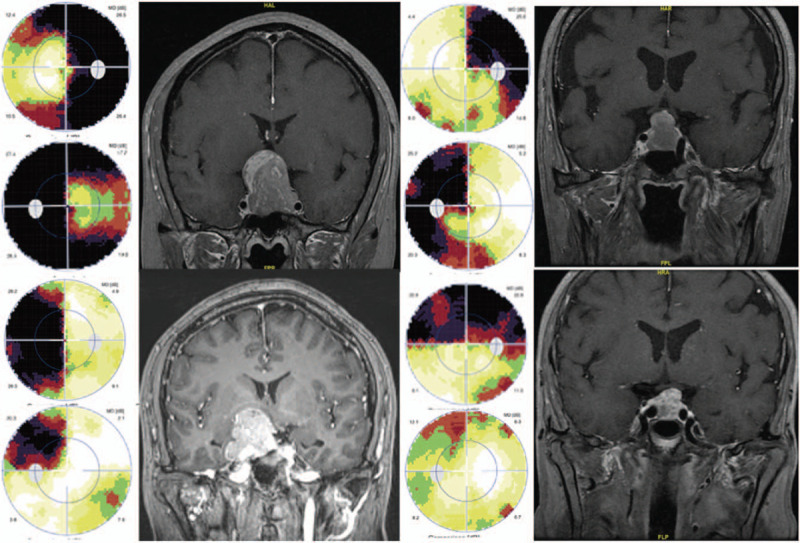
Visual field and MRI of four patients with pituitary adenoma. Top-left: Case 1. A 53-year-old woman with NFPA at diagnosis. Visual field: bilateral temporal hemianopsia breaking through the vertical midline. Contrast-enhanced MRI shows that the pituitary dumbbell-shaped mass with a clear boundary is significantly enhanced, with a size of 2.7 × 2.6 × 4.0 cm. The mass protrudes to the sella and compresses the optic chiasma. Top-right: Case 2. A 70-year-old man with NFPA at diagnosis. Visual field: bilateral superior temporal quadrant visual field defect and breakthrough to the inferior temporal region. Contrast-enhanced MRI shows that the tumor is significantly enhanced, with a size of 1.9 × 1.8 × 2.5 cm and a clear boundary. The pituitary stalk is shifted to the left, and the optic chiasma is displaced upwards. Bottom-left: Case 3. A 47-year-old man with FPA (parasellar prolactinoma) at diagnosis. Visual field: nasal hemianopsia in the right eye and a quadrant visual field defect in the left eye. Contrast-enhanced MRI shows that the tumor is significantly enhanced, with a size of 2.0 × 2.7 × 3.0 cm and an irregular boundary. The pituitary stalk is shifted to the left, and the anterior part of the optic chiasma is obviously elevated on the right side. Bottom-right: Case 4. A 67-year-old man with NFPA at diagnosis. Visual field: superior hemianopsia in the right eye and a superior local defect in the left eye. Contrast-enhanced MRI shows that the tumor is 1.8 × 1.7 × 1.9 cm, and the left optic chiasma is compressed and displaced upwards. MRI = magnetic resonance imaging, FPA = functioning pituitary adenoma, NFPA = non-functioning pituitary adenoma.
